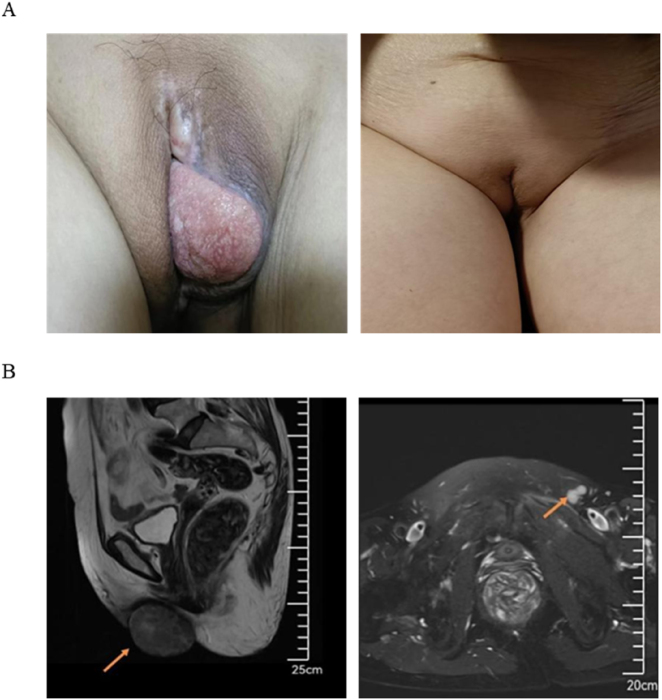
Clinical findings. (A) Preoperative and postoperative comparison of a vulvar cancer patient. (B) The provided MRI image shows the patient’s tumor tissue. Both the tumor mass and the enlarged lymph nodes are indicated with orange arrows.
